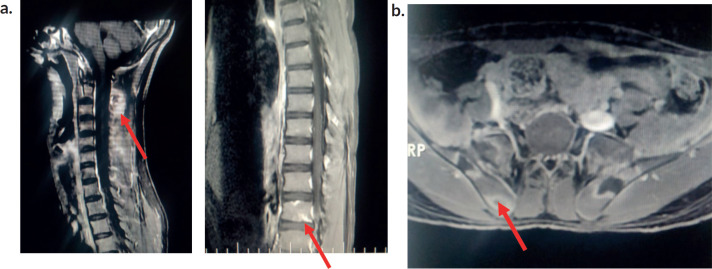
Magnetic resonance imaging of the spine . (a): MRI spine-post contrast enhancement noted in C2 and C6 vertebral bodies.Osteolytic lesion noted at C2. Ill-defined enhancement noted in the paraspinal muscles posterior to C2 and thoracic vertebra. (b): MRI Hip -Ill-defined STIR hyperintense foci noted in bilateral iliac bones and sacrum (ala) with peripheral enhancement.
